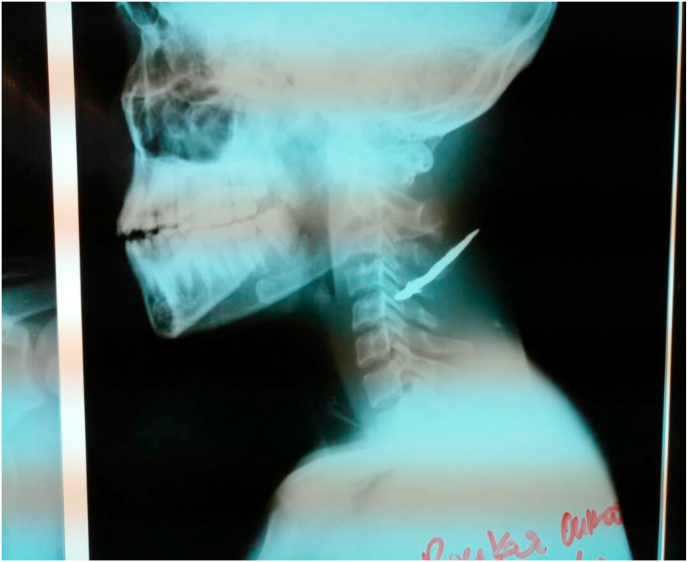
X-ray of the neck of the patient on admission to the emergency room.
